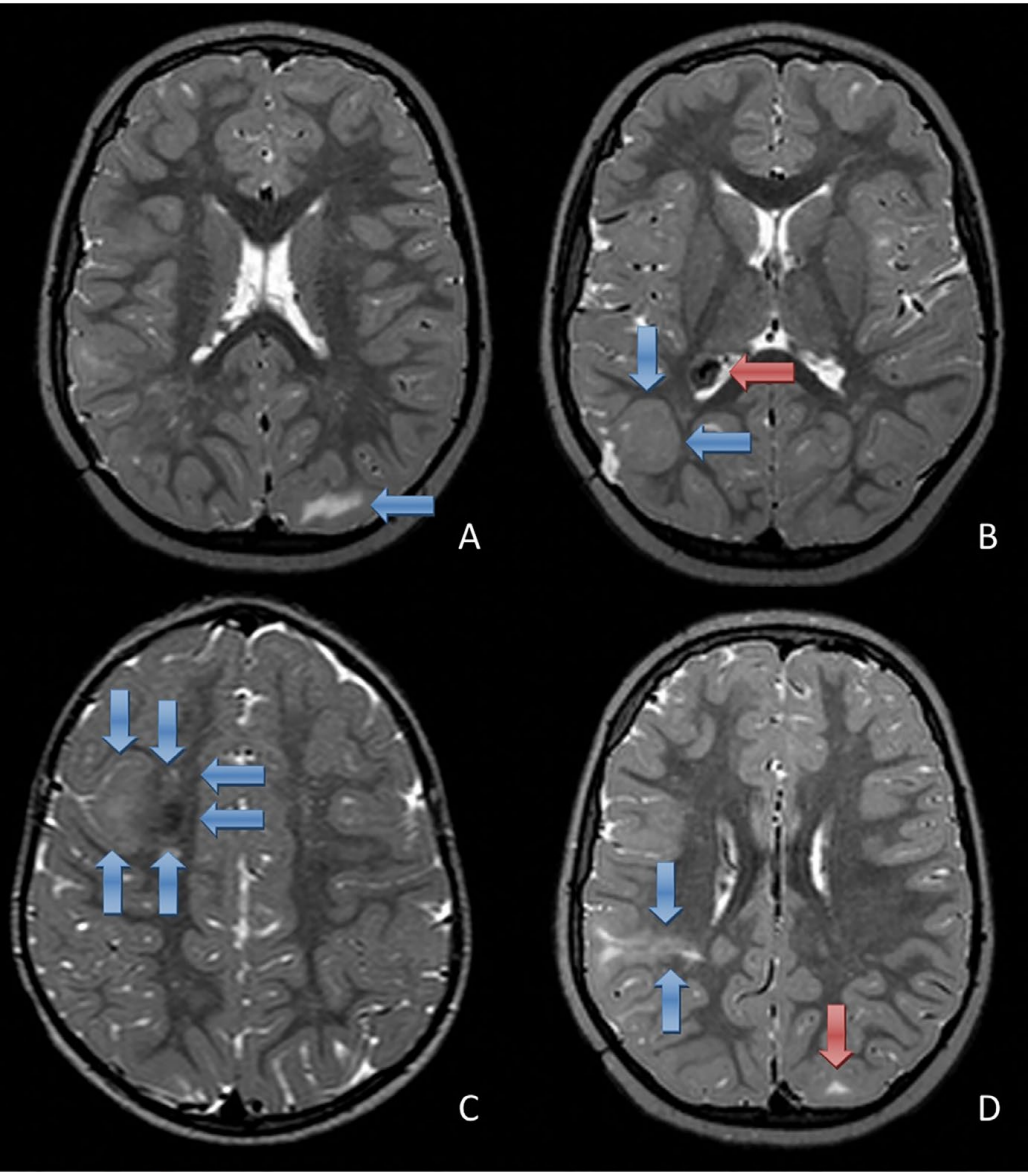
MRI abnormalities in TSC, shown on T2 weighted images. Tuber in the left occipital lobe, seen as a hyperintense lesion (blue arrow, A). Increased cortical thickness in the right parieto‐occipital region (blue arrows, B). Also, a SEGA is shown in the lateral ventricle, unrelated to patient's epilepsy (red arrows, B). Blurring of the gray‐white matter junction with a calcification in the right frontal lobe (blue arrows, C). Transmantle sign in the right parietal lobe, seen as a subcortical hyperintense lesion with a tail extending toward the ventricle (blue arrows, D). Also, a tuber is shown in the left occipital lobe, seen as a small subcortical hyperintense lesion (red arrow, D)
